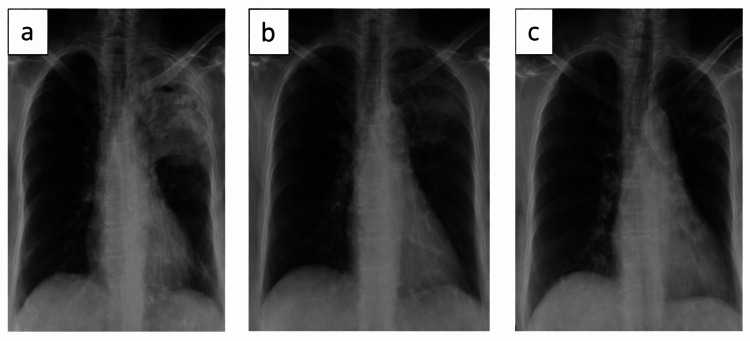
Chest roentgenogram at baseline (a), two months (b), and at the end of actinomycosis treatment (c)
